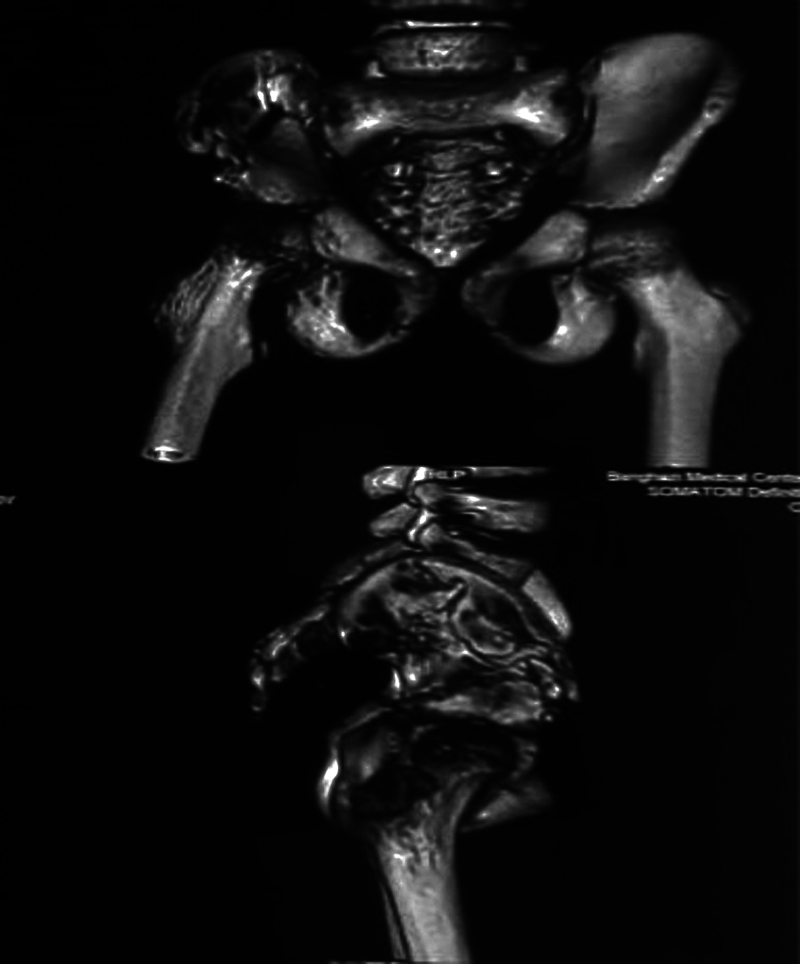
Computed tomography 3D reconstruction in a child with GSD showing osteolysis of the right proximal femur and iliac crest.
